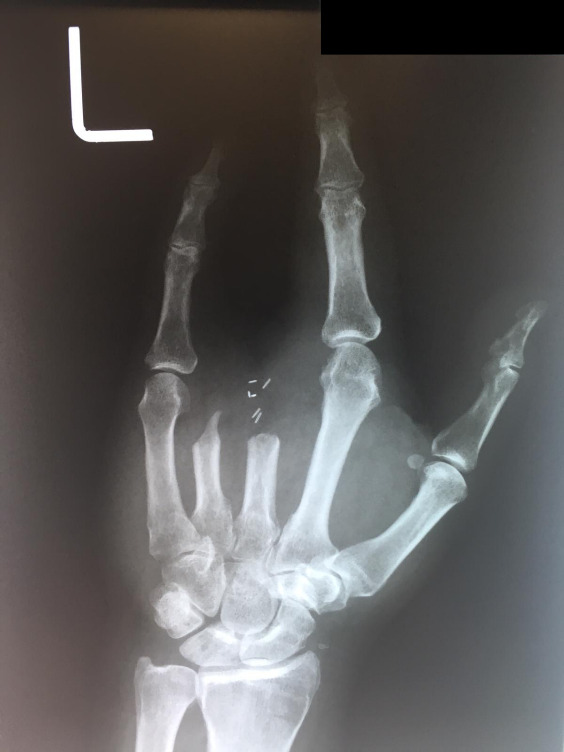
PA radiograph of the left hand after the second surgery but prior to the third surgery, demonstrating partial amputation of the third and fourth metacarpals.
